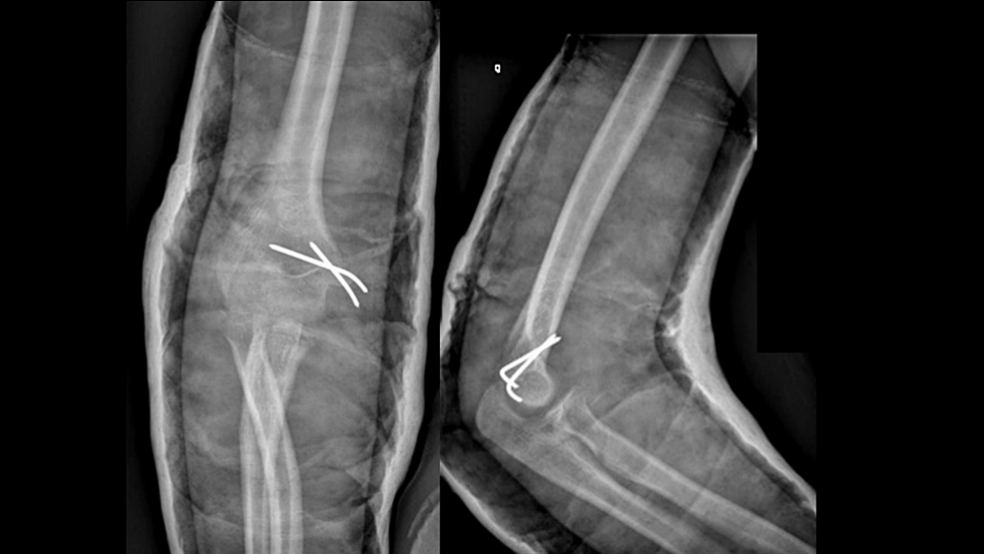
Radiographs of the elbow at the six-week follow-up showing a well-reduced elbow and stable fixation of the medial epicondyle.
